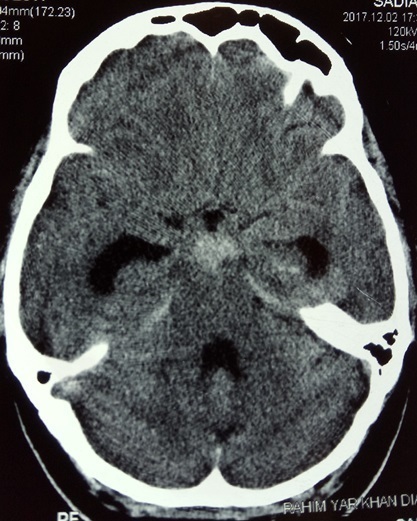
CT scan brain obtained 1 year back showing subarachnoid hemorrhage in interpeduncular cistern.
